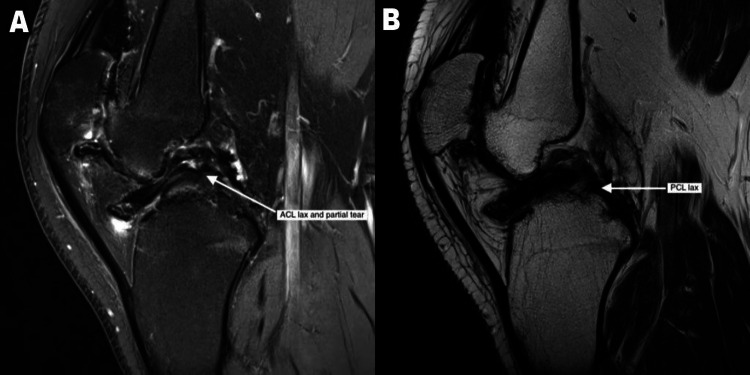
ACL showing lax and partial tear and PCL showing lax (A) ACL, MRI image showing lax and partial tear; (B) PCL, MRI image showing lax. ACL: anterior cruciate ligament; PCL: posterior cruciate ligament.
